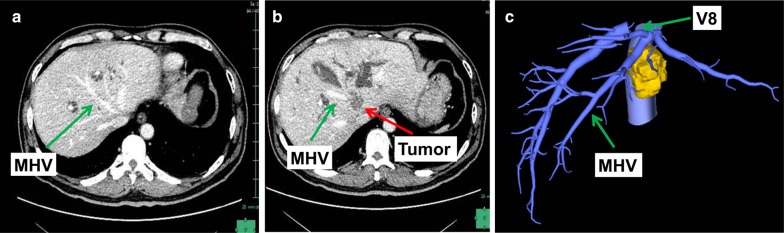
Relationship between tumor and hepatic veins: a and b are CT scan which shows that the tumor infiltrated the root of the middle hepatic vein, and the left hepatic vein was not infiltrated. c The three-dimensional reconstruction of the tumor and hepatic vein. *MHV* middle hepatic vein, *V8* hepatic vein of segment 8
